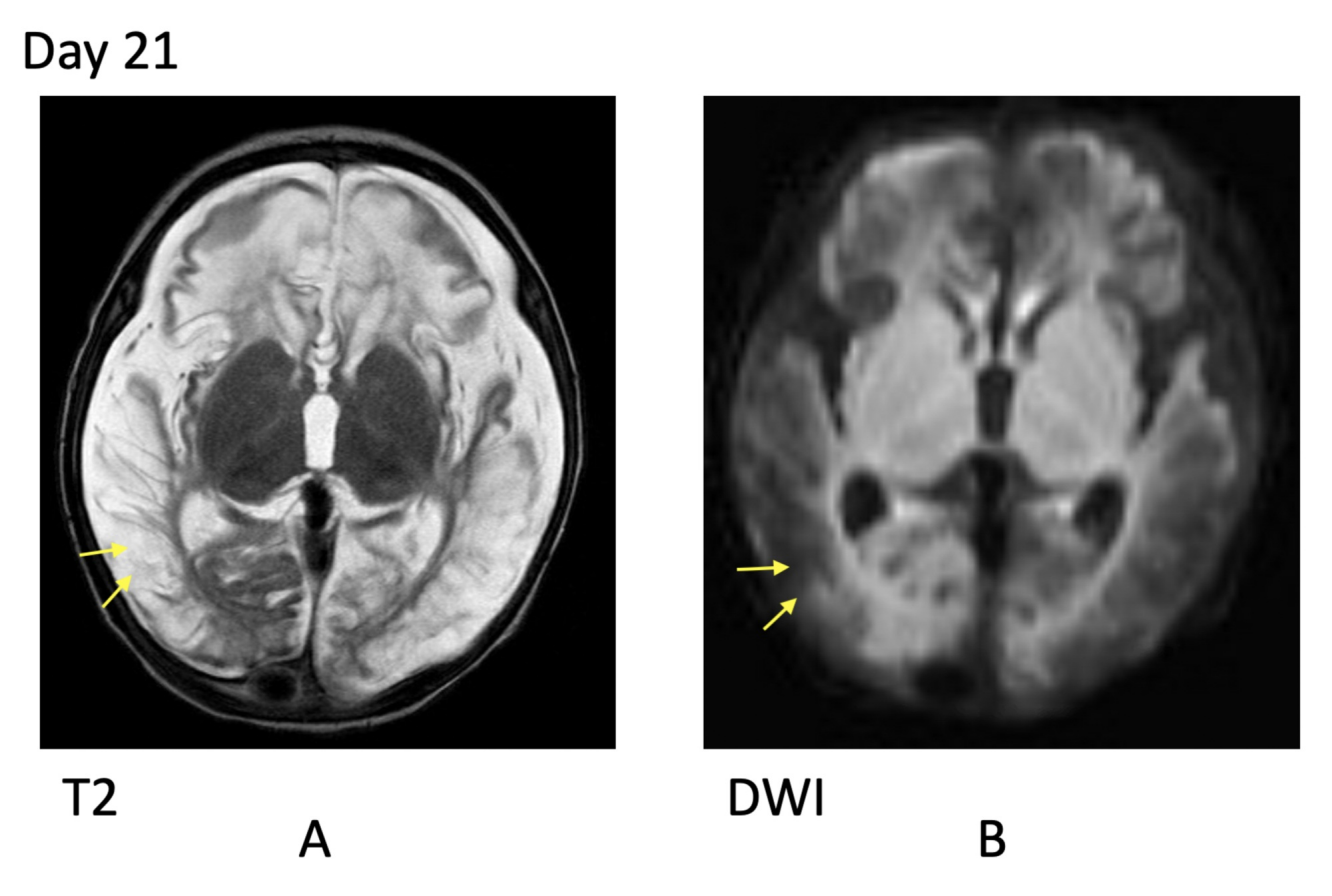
Radiological findings on day 21 of admission A: MRI T2 showing severe diffuse cortical atrophy, including the occipital cortex; B: MRI DWI demonstrates abnormal (facilitated) cortical diffusion, appearing dark, consistent with diffuse cortical encephalomalacia MRI: magnetic resonance imaging; DWI: diffusion-weighted imaging
